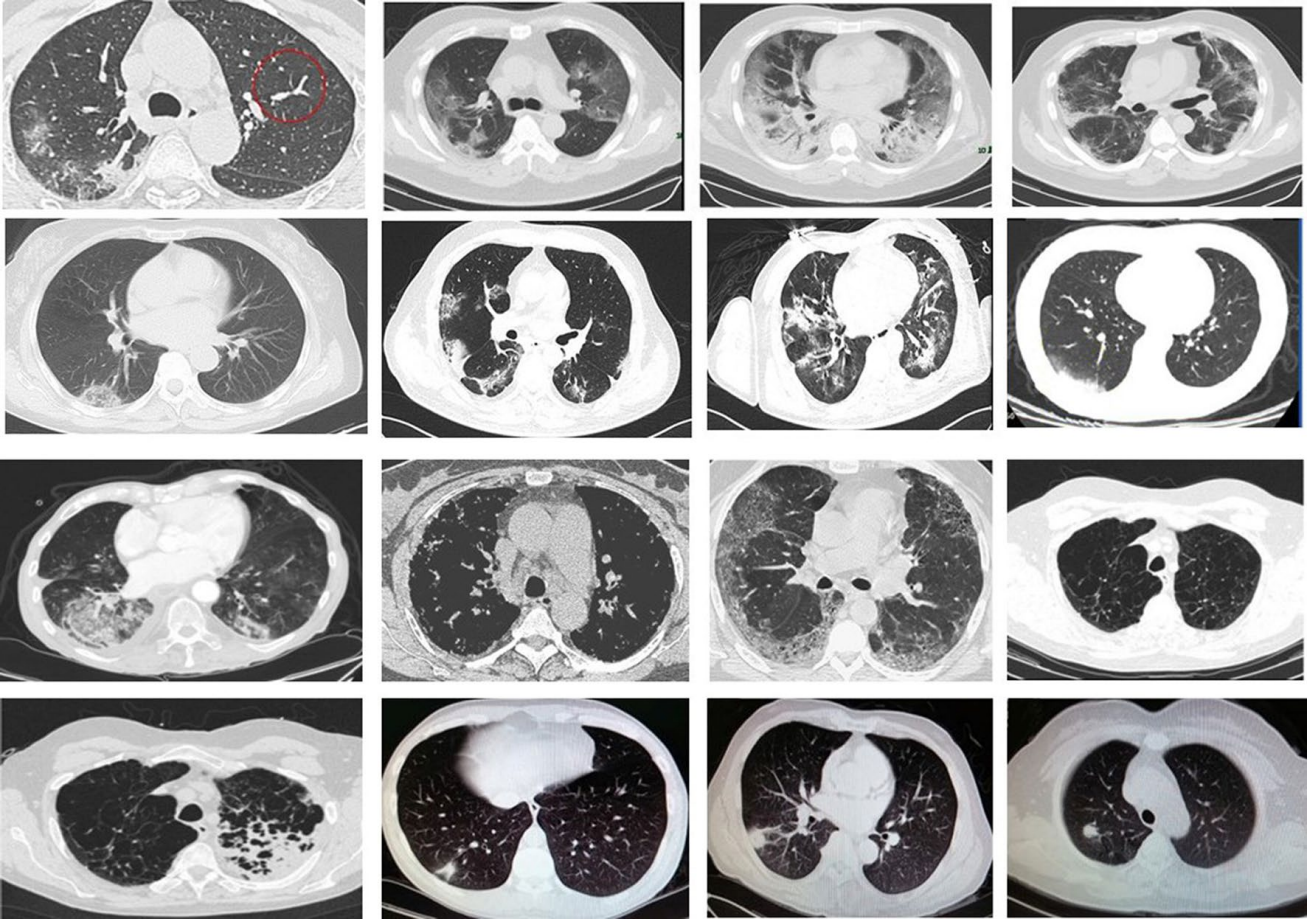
CT images: Rows 1 and 2: COVID-19, Rows 3 and 4: non-COVID-19 (aspect ratios of some images were rescaled to fit the figure frame).
